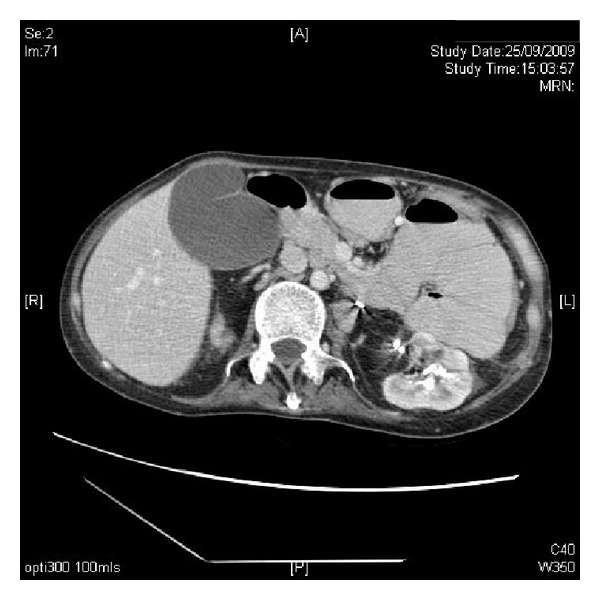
CT abdomen, performed on 25 September 2009, revealed loops of dilated proximal small bowel and distended gall bladder.
